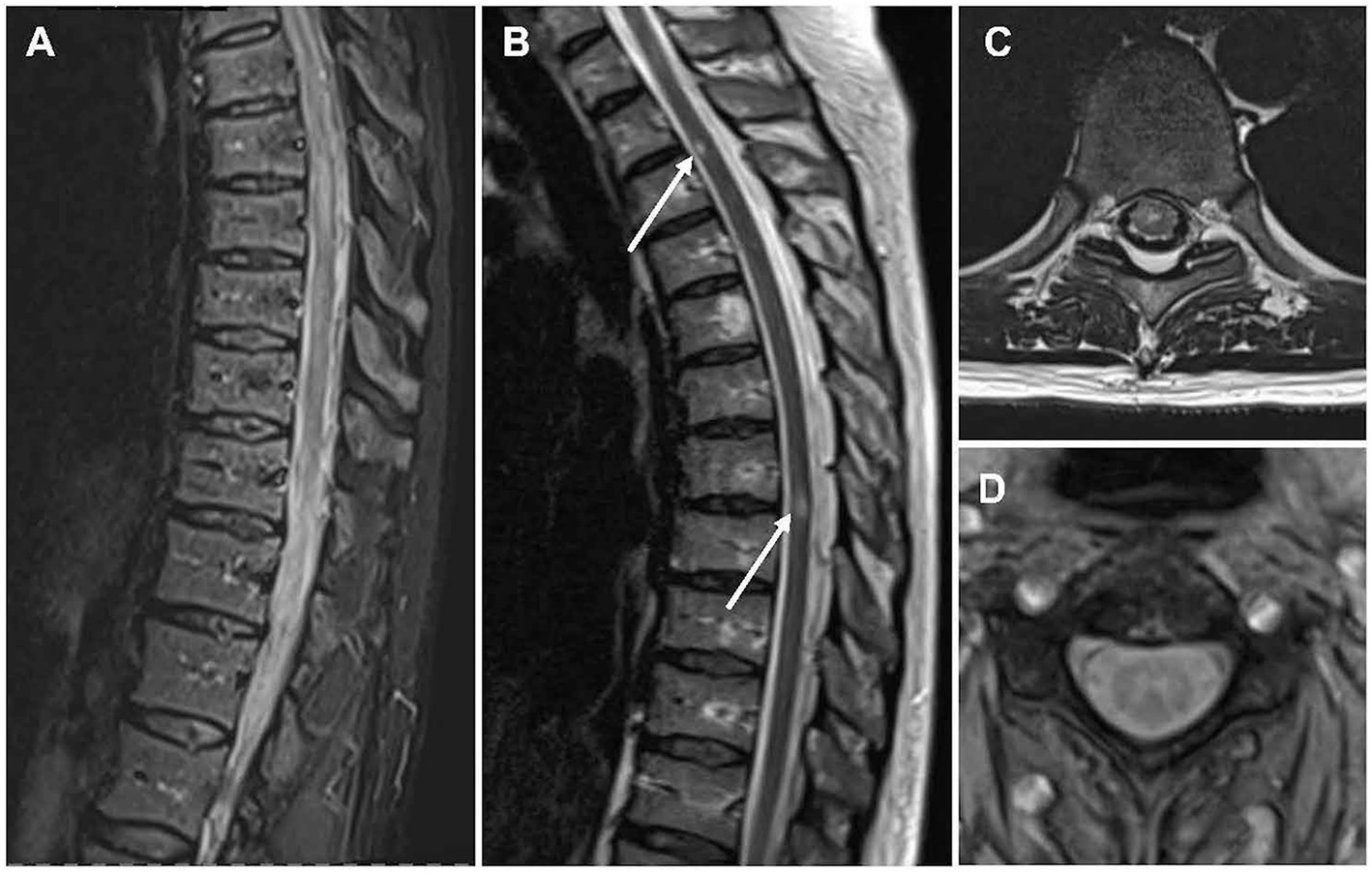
MR imaging of the spinal cord in people with MOGAD. Sagittal T2-weighted MRI showing hyperintense lesions in line with (A) an LETM and (B) shorter lesions. Axial T2-weighted MRI showing (C) a centrally located lesion and (D) the characteristic H-sign. LETM, longitudinally extensive transverse myelitis; MOGAD, myelin oligodendrocyte glycoprotein antibody associated disease; MRI, magnetic resonance imaging.
